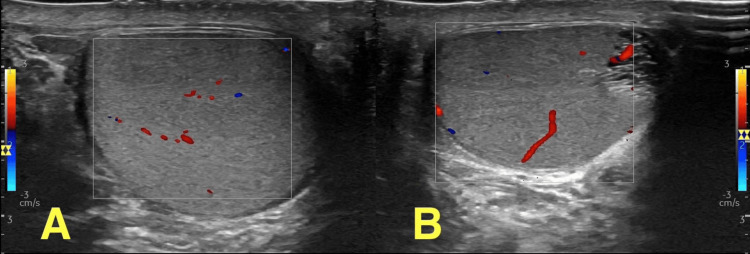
Doppler ultrasound Doppler ultrasound shows preserved blood flow in the left (unaffected) testis, despite the presence of testicular atrophy, which is presumed to have been preexisting. These findings highlight the importance of testicular vessel repair in young patients due to the potential risks involved (A, right testis; B, left testis)
